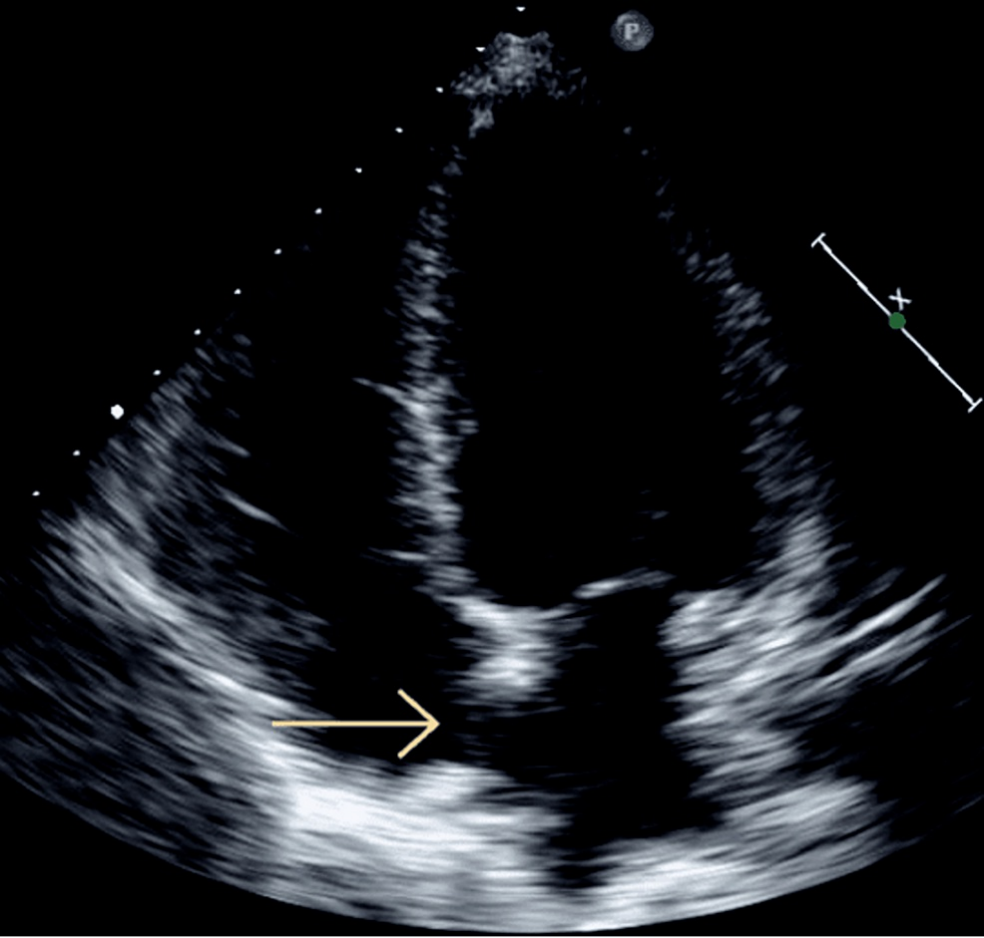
Transthoracic echocardiography showing large patent foramen ovale (pale yellow arrow)
